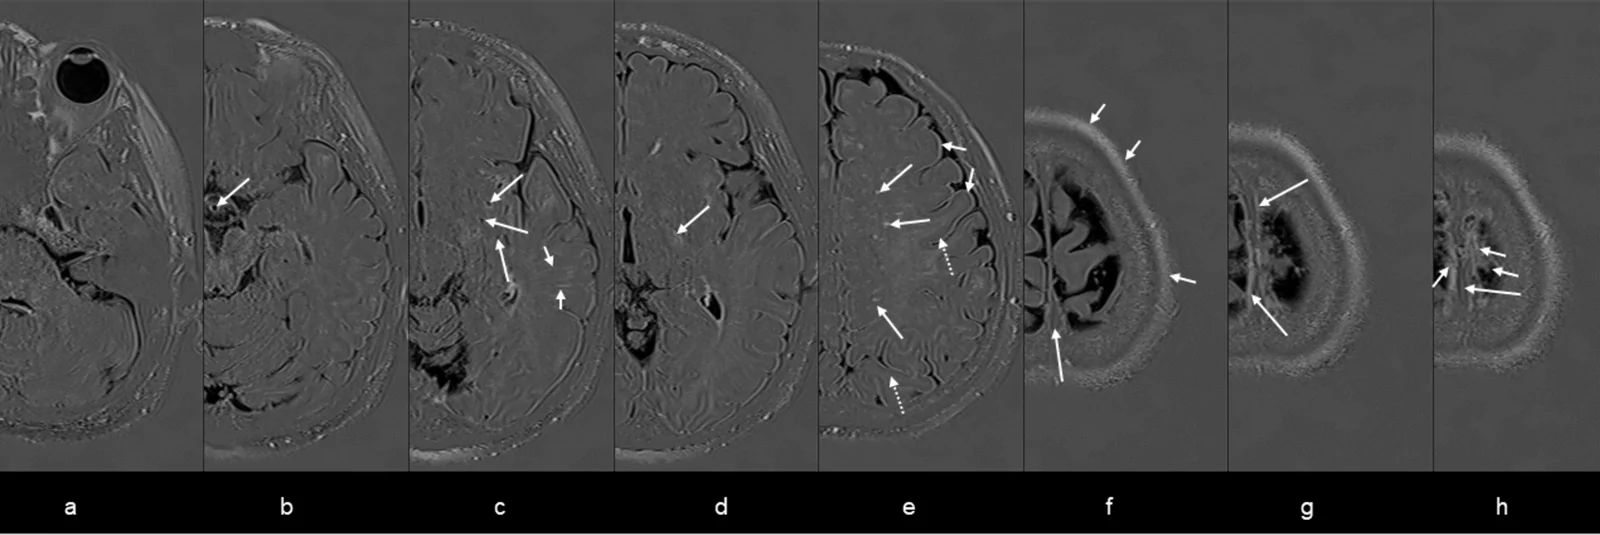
A woman in her 40 s who underwent MR imaging for dizziness, with no evidence of endolymphatic hydrops. Non‑contrast PRISM brain images are shown from the skull base to the vertex. a At the level of the internal auditory canal, the anterior chamber and vitreous body of the eyeball are depicted as hypointense and appear black. The inner ear structures and cerebrospinal fluid (CSF) in the cerebellopontine angle are also hypointense and appear dark. b At the level of the interpeduncular cistern, CSF appears black, and the cerebral aqueduct is also depicted as hypointense. The infundibular recess shows a dark signal within its lumen (arrow). The brain parenchyma, including both gray matter and white matter, is depicted as nearly uniform gray. c At the level of the basal ganglia, perivascular spaces within the basal ganglia are depicted as hypointense and appear black (arrows). In contrast, perivascular spaces in the cerebral white matter of the temporal lobe appear hyperintense (short arrows). The choroid plexus in the trigone of the lateral ventricle is depicted as hyperintense. d At the level of the posterior limb of the internal capsule, the corticospinal tract is depicted as faintly hyperintense (arrow). e At the level of the centrum semiovale, white matter lesions are depicted as hyperintense (arrows). The superficial layer of the cerebral cortex appears as if covered by a faint, thin hyperintense membrane (short arrows). In regions where the white matter signal is relatively high, the gray matter appears slightly hypointense in comparison (dotted arrows). f At the level of the inferior margin of the superior sagittal sinus, the venous lumen appears gray (arrow). Residual incomplete fat suppression is observed in the subcutaneous fat at the vertex (short arrows). g On the slice immediately superior to (f), structures presumed to represent meningeal lymphatic vessels along the superior sagittal sinus are depicted as hyperintense (arrows). h On the most superior slice, the parasagittal dura is depicted as faintly hyperintense (short arrows). A faint linear hyperintense structure within the lumen center of the superior sagittal sinus is presumed to represent the chordae Willisii (arrow). PRISM, Polarity-preserving Real Inversion Solute Mapping
